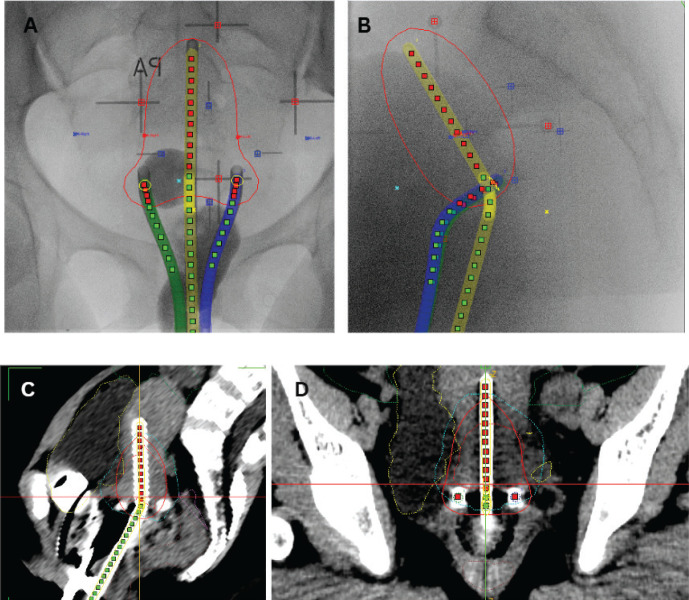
Cervical cancer BT techniques*. (a)*: AP-PA view of* X*-ray image for 2D point A based BT planning.* (b)*: Sagittal view of* X*-ray image for 2D point A based BT planning.* (c)*: Sagittal view of CT-based 3D BT planning.* (d): AP-PA view of CT-based 3D BT planning.
